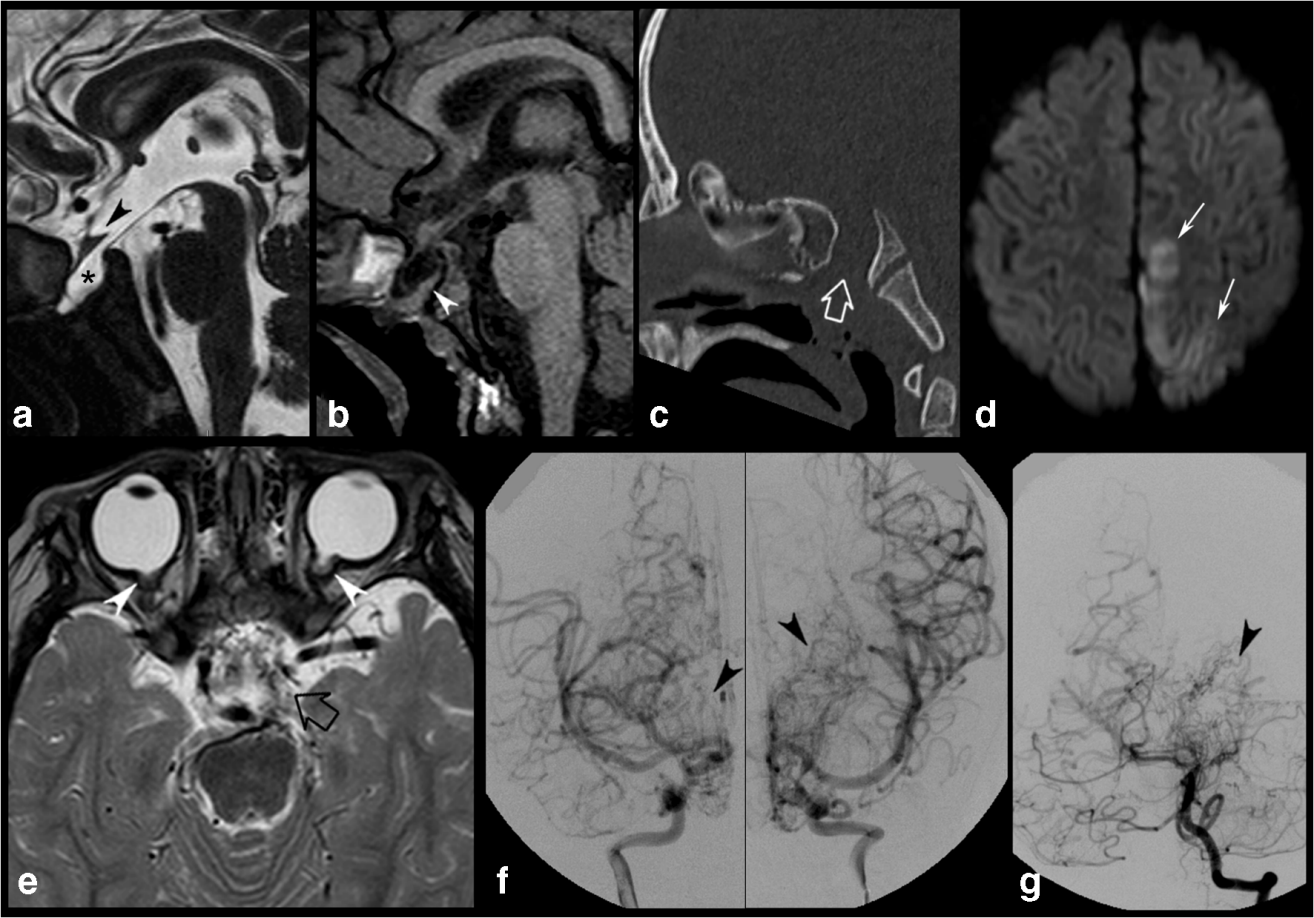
Preoperative imaging findings of the patient. a, b Brain MRI and c CT scan performed at 6 months of age. d, e Brain MRI. f, g Digital subtraction Aagiography performed at 2 years of age. Sagittal T2 heavily weighted (DRIVE) (a) and T1-weighted (b) images reveal a spheno-nasopharyngeal cephalocele with herniation of the pituitary gland and third ventricle through a sphenoid bone defect. The pituitary gland is flattened against the clivus (arrowhead, b). The optic chiasm is distorted and displaced downward (arrowhead, a). c 3D CT scan, sagittal MPR reconstruction demonstrates a large craniopharyngeal canal (empty arrow). d Axial diffusion-weighted imaging performed at 2 years of age demonstrates an acute ischemic infarct in the left medial parietal and frontal lobes (thin arrows). e Axial T2-weighted image shows multiple tiny flow voids in the basal cisterns consistent with moyamoya vascular collaterals (empty arrow). Note the bilateral optic nerve funnel-shaped excavations with associated abnormal soft tissue and discontinuity of the normal uveoscleral coat at the optic nerve insertions, in keeping with MGDA (arrowheads). Preoperative digital subtraction angiography, frontal views, internal carotid artery injections (f) and left vertebral artery injection (g) confirm the diagnosis of moyamoya disease with bilateral stenosis of the supraclinoid carotid arteries, anterior cerebral arteries, and left posterior cerebral artery, and the typical “puff of smoke” sign due to the presence of compensating vascular networks (arrowheads)
